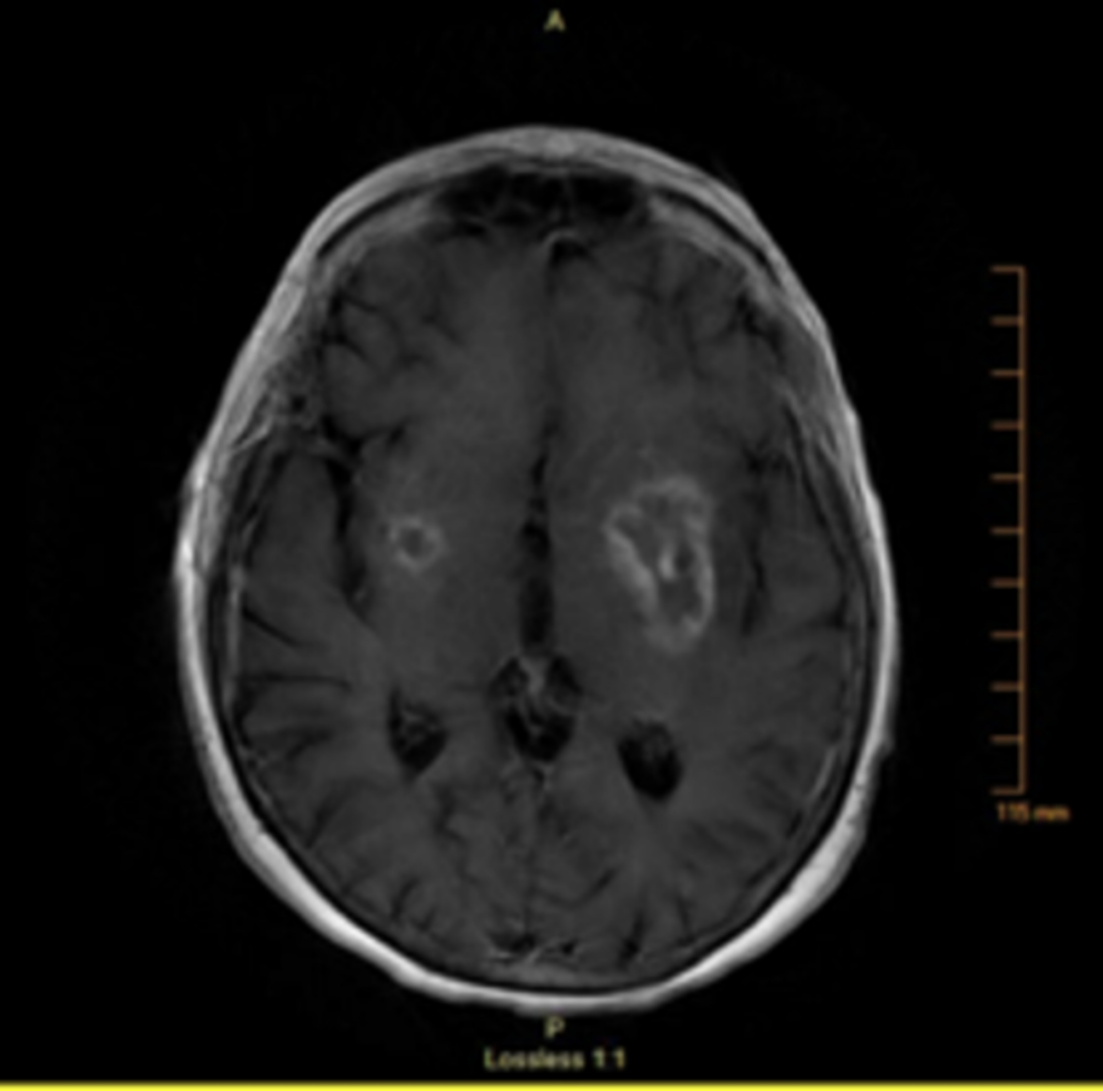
MRI of the brain (T1 fluid-attenuated inversion recovery) - view 1 The imaging revealed a 4 x 2 cm ring-enhancing in the basal ganglia on the left with a small enhancing lesion in the caudate nucleus as well as a small 1 cm round ring-enhancing lesion in the putamen on the right. There were surrounding vasogenic edema and mild mass effect on the left lateral ventricle MRI: magnetic resonance imaging
